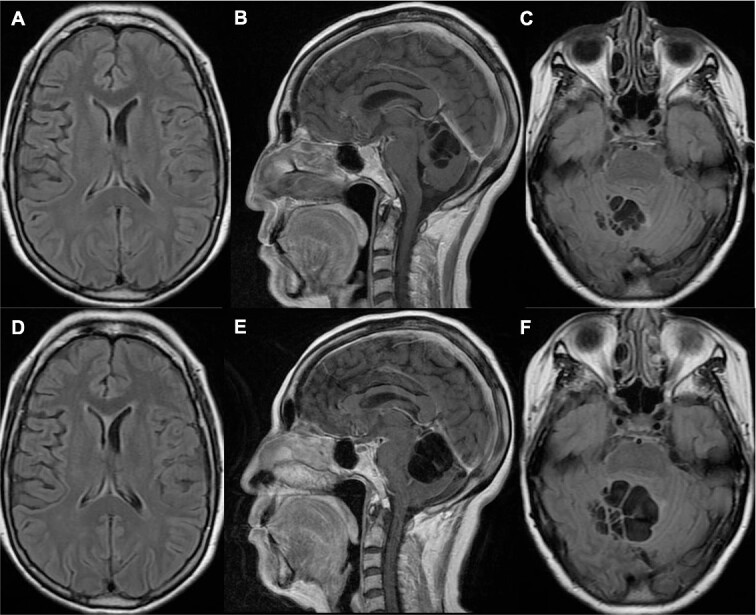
Brain MRI one year (A–C) and 14 months (D–F) prior to her current admission. (A–B) T1 sequence depicted multiple cystic lesions in the cerebellum without significant compression of the fourth ventricle; (C) T2 fluid-attenuated inversion recovery (FLAIR) sequence showed slight perilesional edema; (D–E) T1 sequence demonstrated a notable increase in cyst growth along with compression of the fourth ventricle; (F) T2 FLAIR sequence showed increased edema.
